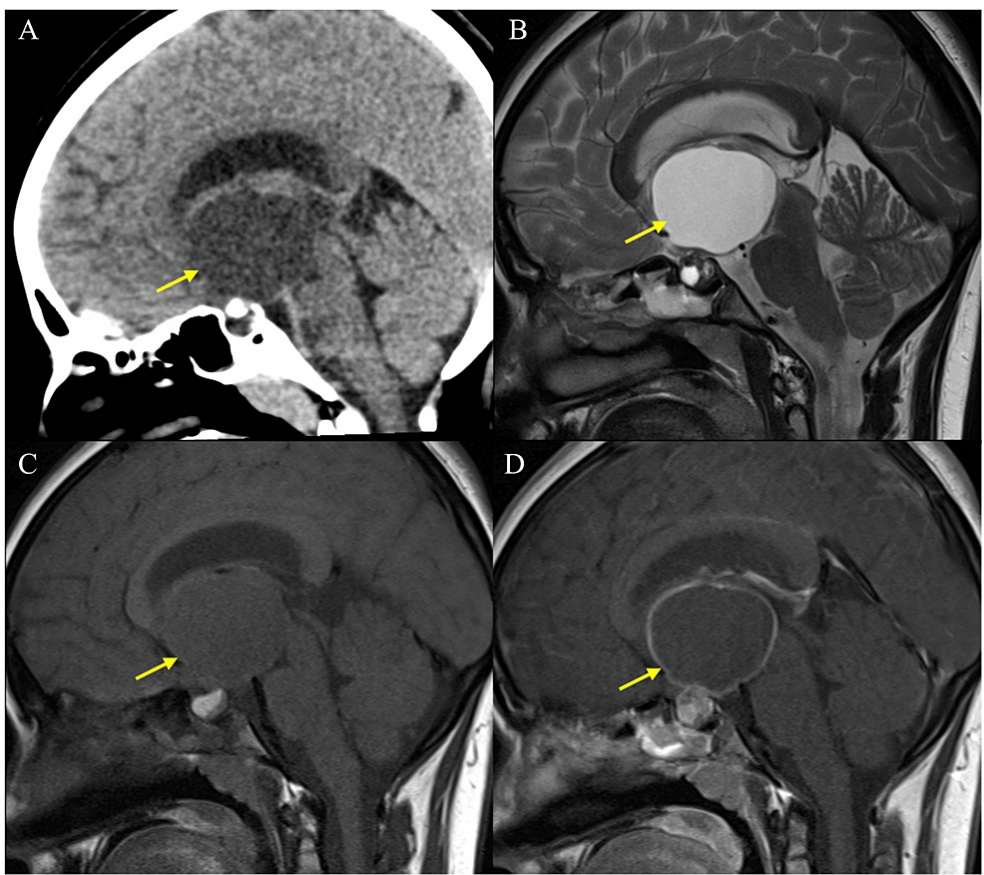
The unenhanced CT of the brain (A) demonstrates a sellar and suprasellar solid and cystic mass with coarse calcification (yellow arrow). The MRI of the sella shows a predominantly high T2 signal intensity cyst (B) (yellow arrow), which is of iso-signal intensity (to grey matter) on T1(C) (yellow arrow). Enhancement of the solid component and the cyst’s wall is noted on the enhanced T1 image (D) (yellow arrow). All the features indicate a diagnosis of an adamantinomatous craniopharyngioma.
